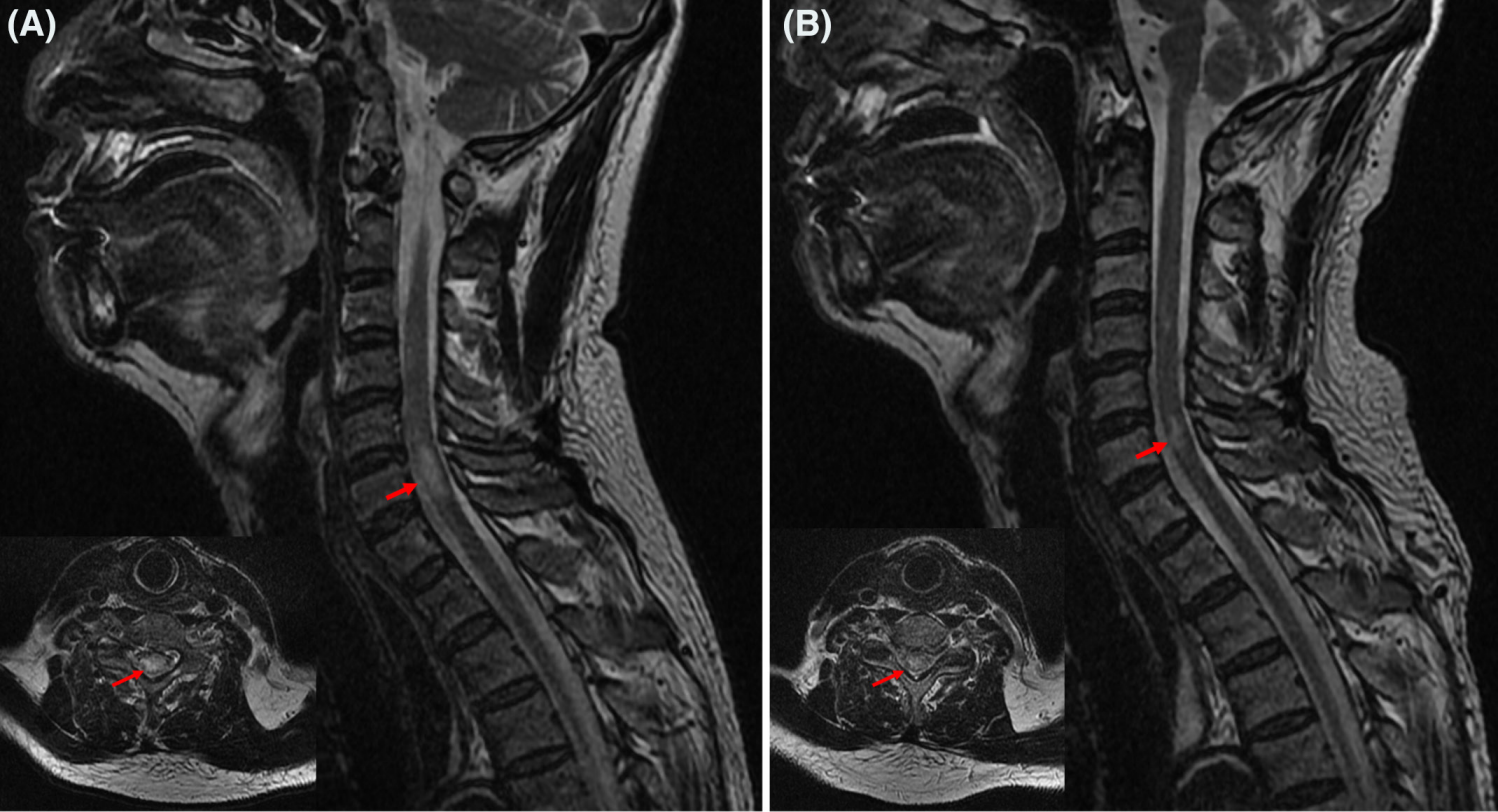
Cervical spine MRI. (A) T2‐weighted MRI of the cervical spine on day 9 presented an intramedullary high signal. (B) T2‐weighted MRI of the cervical spine on day 34 showed a significantly absorbed abnormal signal. (magnetic resonance imaging, MRI)
